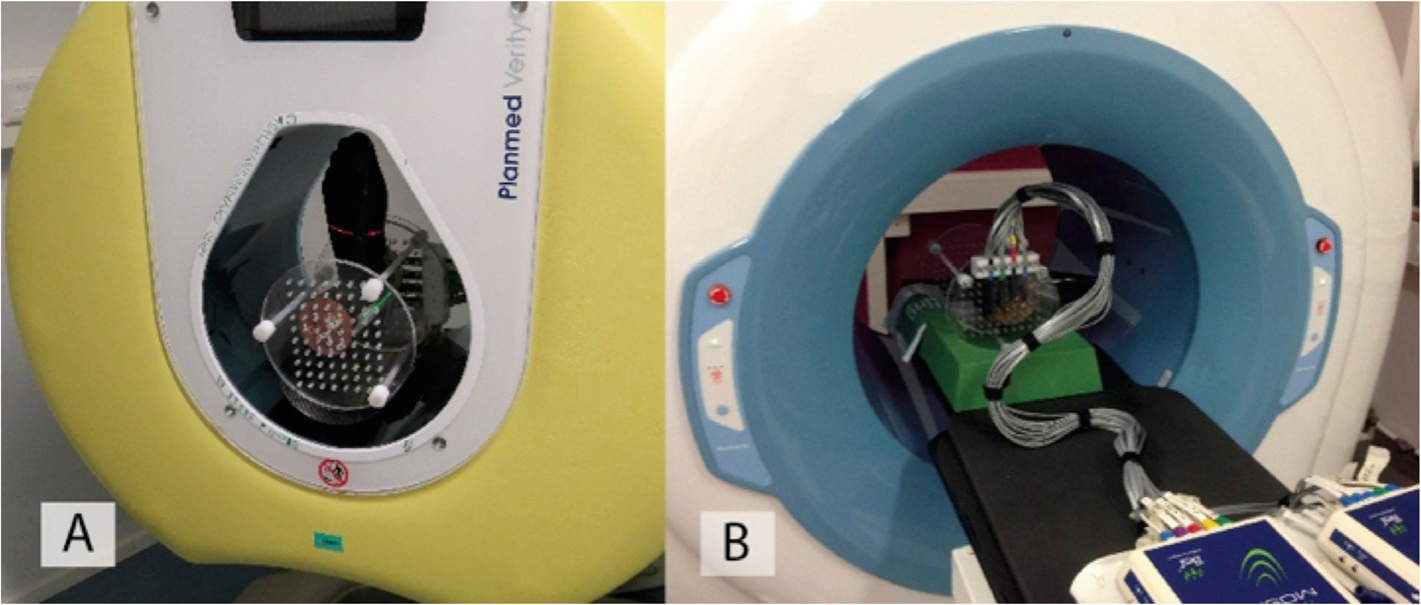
The measurement setup for the Planmed Verity CBCT scanner (A) and the NewTom 5G CBCT scanner (B).
